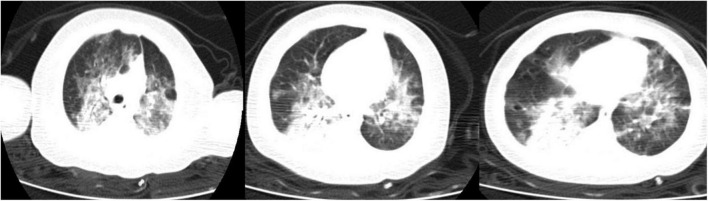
Chest Computed tomography (CT) scan of the patient showing an interstitial pneumonia.
